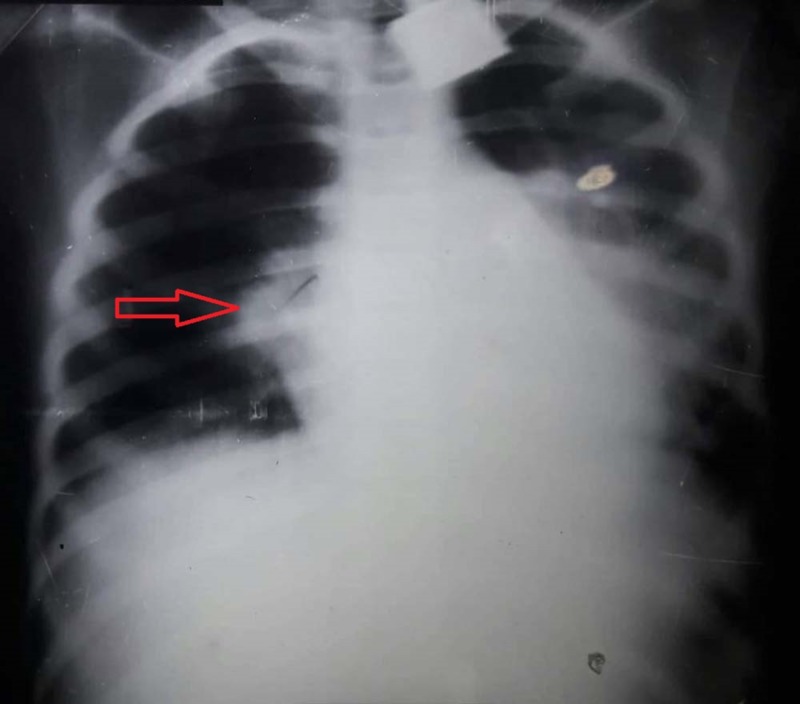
X-ray chest showing right-sided lobar pneumonia
